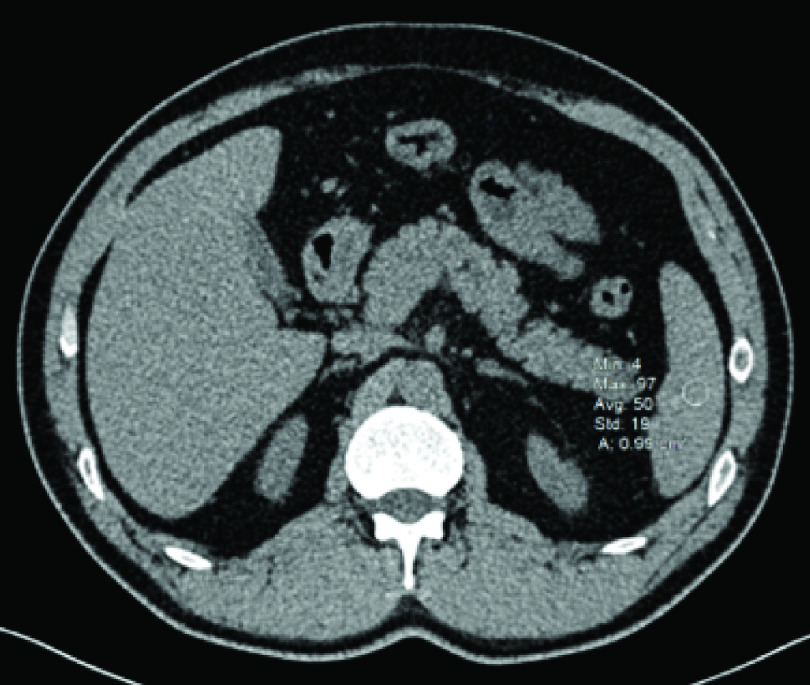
Axial computed tomography image of the same patient. Three ROIs (using a circular ROI of 1 cm^2^) were drawn from the upper, middle and lower parts of the spleen, to assess the attenuation value of the organ by taking the average of the three HU values collected from the ROIs. Shown here is the measurement from the lower a third of the spleen (50 HU). The average attenuation value was 51 HU in the patient. By dividing the attenuation value of the pancreas with the attenuation value of the spleen, a pancreas-to-spleen attenuation ratio of 0.68 was found, which was smaller than 0.70 (cut-off ratio of pancreas-to-spleen attenuation for pancreatic steatosis), confirming the diagnosis of pancreatic steatosis in this patient. HU: Hounsfield unit; ROI: Region of interest.
